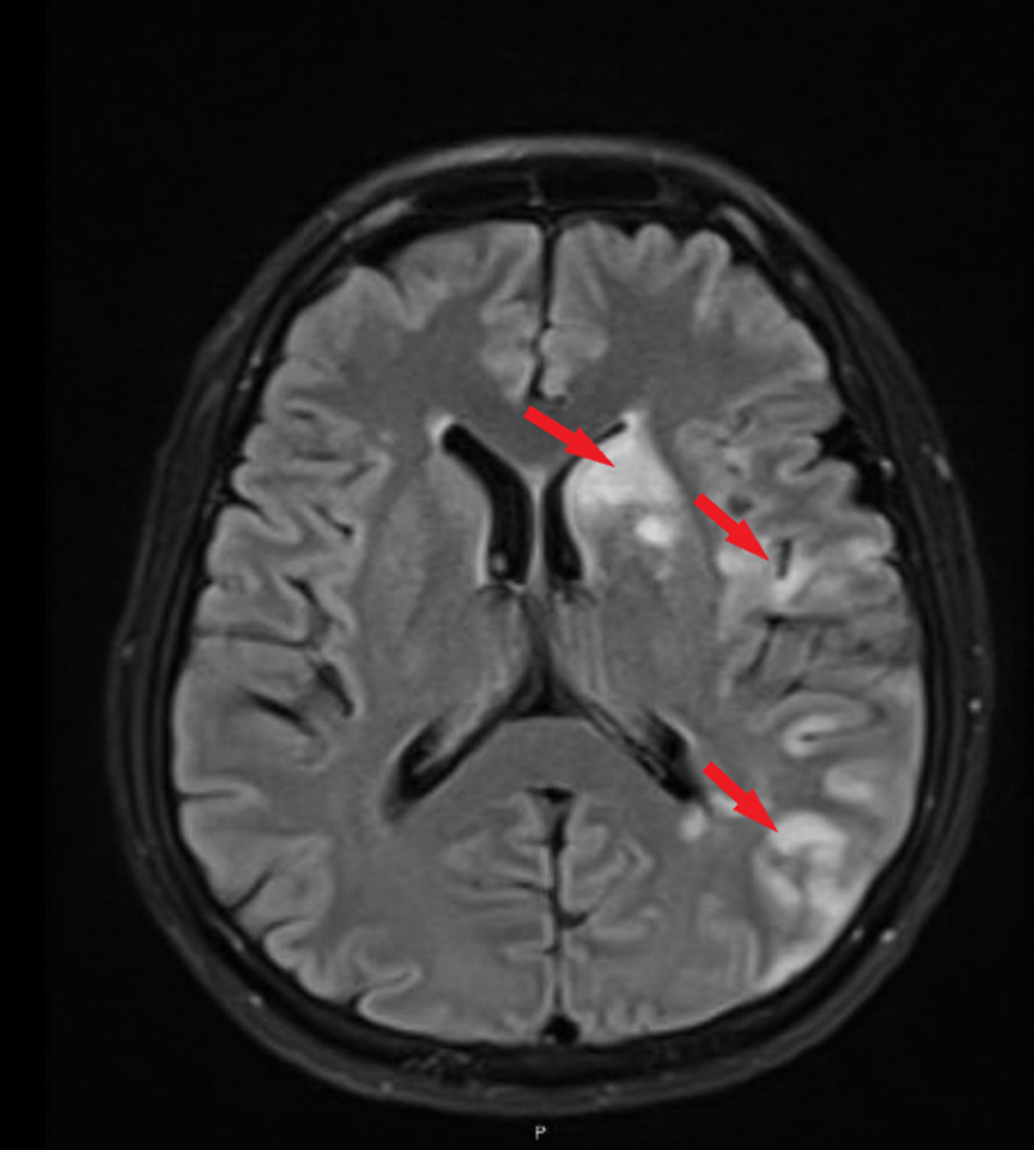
MRI Head showing multiple acute ischemic infarctions (arrows) in left basal ganglia and left cerebral hemisphere.
